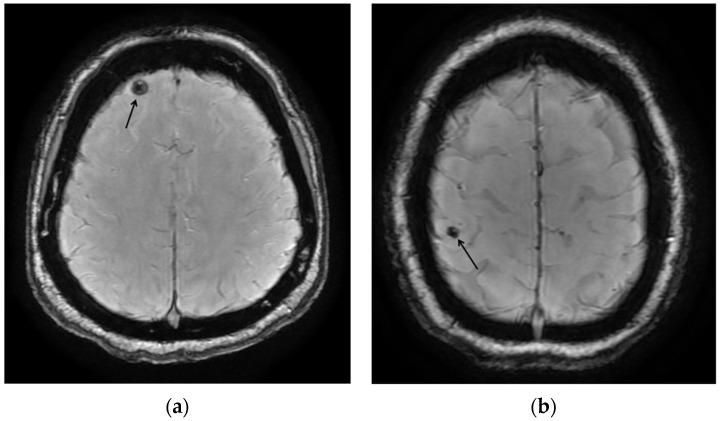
MRI images: (a) the susceptibility-weighted image shows a round hypointense calcification (arrow) in the right anterior frontal lobe, with no surrounding edema or mass effect, consistent with the calcified nodular stage of NCC; (b) the susceptibility-weighted image shows a round hypointense calcification (arrow) in the right superior parietal lobule, with no surrounding edema or mass effect, consistent with the calcified nodular stage of NCC.
